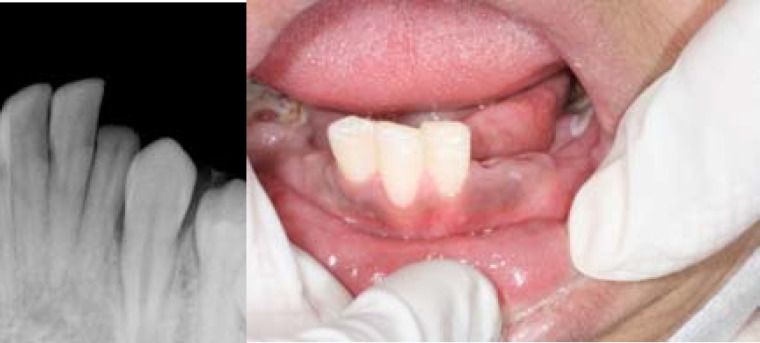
Clinical and radiographic view of fractured incisore
